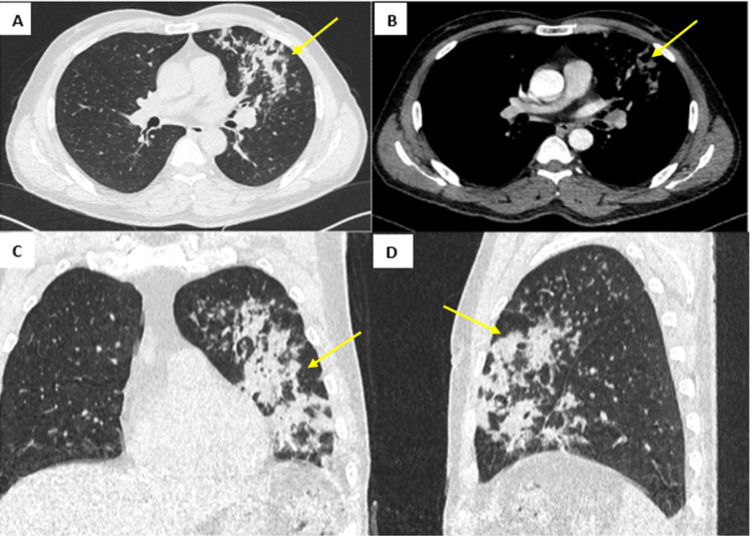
Computed tomography scan of chest with intravenous contrast, lung window A) axial, C) coronal and D) sagittal cuts, showing patchy areas of consolidation in the left lung. B) Axial soft tissue window showing focal lesions with central non-enhancing hypodensity, suggestive of low-density consolidation in the left hilum.
